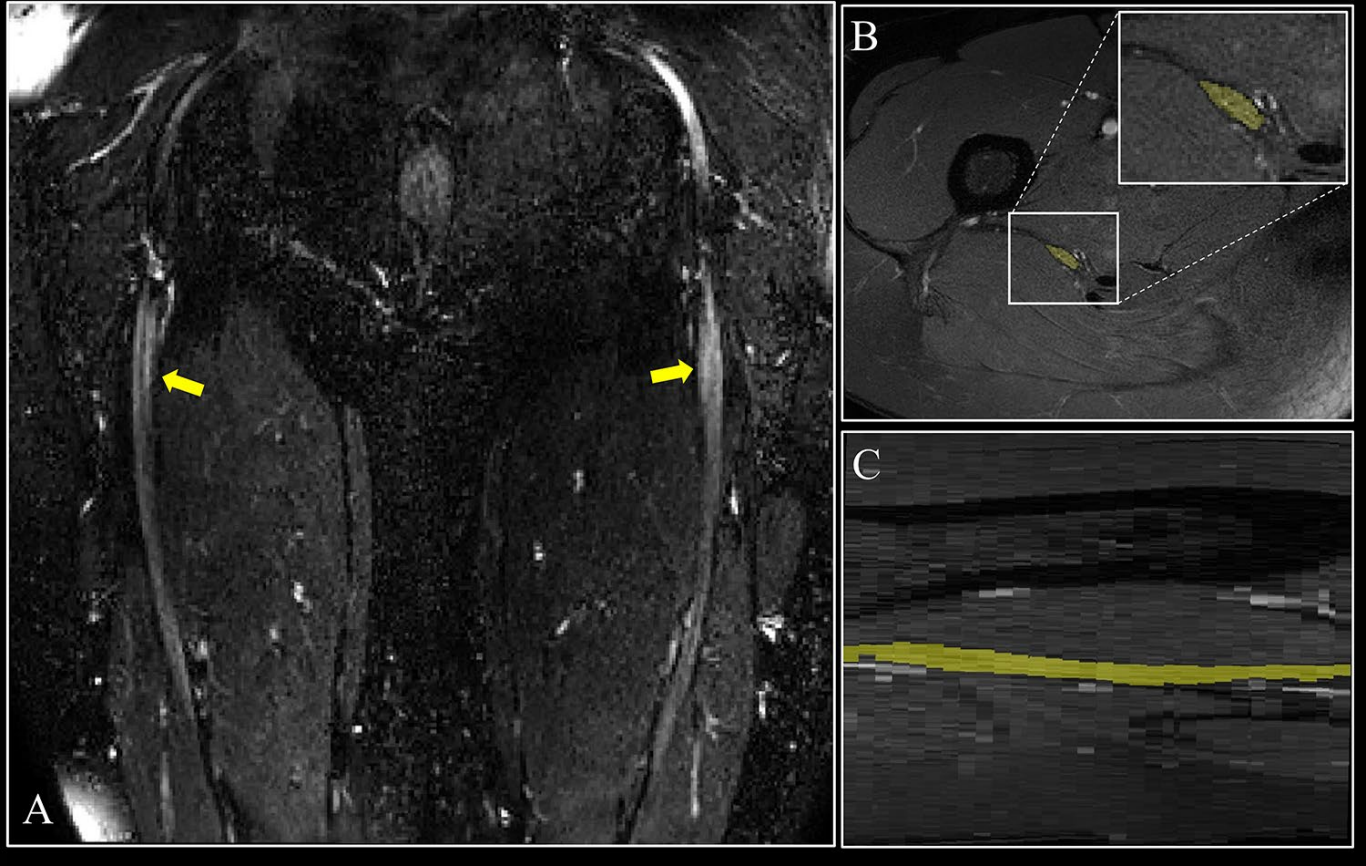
(A) Large field-of-view 3D SHINKEI image in the coronal plane showing the sciatic nerves (yellow arrows) in a healthy control; (B) Manual segmentation of the sciatic nerve slice-by-slice in the axial plane using the fat-suppressed T2-weighted acquisition (binary mask shown in yellow); (C) Segmentation of the sciatic nerve shown in the sagittal plane (binary mask shown in yellow).
